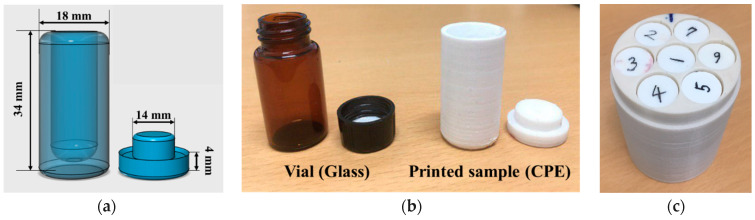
(a) Design showing the test sample, which had the same dimensions as the reference glass vial. (b) Photograph of the glass vial and one of the printed samples. (c) Frame structure and inserted samples for MRI. (1: 1Gy, 2: 2Gy, 3: 5Gy, 4: 7Gy, 5: 10Gy, 6: 15Gy, 7: 20Gy).
